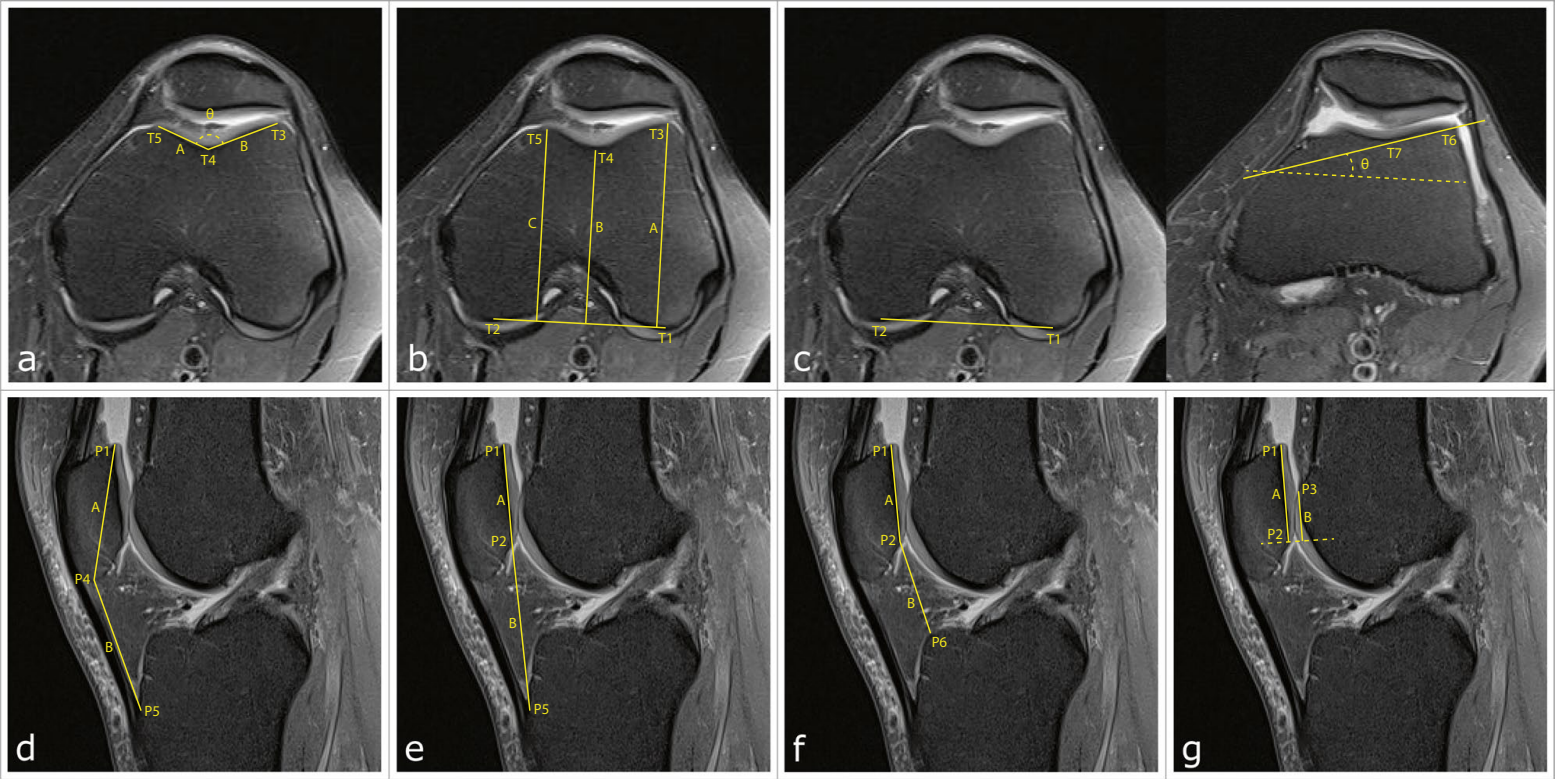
Axial and sagittal fat-saturated proton density weighted turbo spin-echo magnetic resonance images demonstrating the measurements of the indices to assess trochlear dysplasia and patellar height, respectively. The axial slice 3 cm above the femorotibial joint line that includes the intercondylar groove with an appearance of a Roman arch was selected to measure sulcus angle (θ) and trochlear facet asymmetry (A/B) (a), and trochlear groove depth ((A + C)/2-B) (b). The first craniocaudal image showing trochlear cartilage was also selected for measuring lateral trochlear inclination (θ) (c). The sagittal slice showing the longest axis of the patella was selected to measure Insall-Salvati index (B/A) (d), modified Insall-Salvati index (B/A) (e), Caton Deschamps index (B/A) (f) and patellotrochlear index (B/A) (g)
